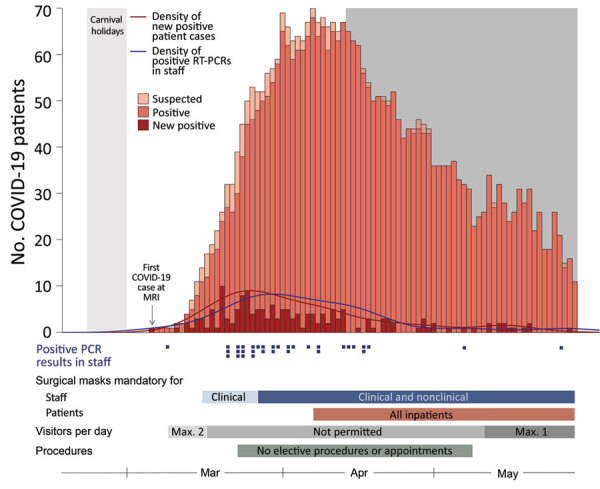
Prevalence and distribution of severe acute respiratory syndrome coronavirus 2 (SARS-CoV-2) infections in patients and staff at a university hospital in Munich, Germany. Shown is the number of all COVID-19 patients admitted to the hospital; the first COVID-19 patient was admitted on March 6, 2020. Light gray shading indicates dates of carnival holidays (February 22‒March 1, 2020); dark gray shading indicates dates of seroprevalence study (April 14‒May 29, 2020). Blue squares below graph indicate positive RT-PCR test results for SARS-CoV-2 RNA in university hospital staff. Bars below graph indicate densities of positive RT-PCR test results in staff (blue), new COVID-19 cases in patients (red), and limitations on number of visitors allowed and elective procedures and appointments (gray). COVID-19, coronavirus disease; Max., maximum; MRI, University Hospital Munich Rechts der Isar; RT-PCR, reverse transcription PCR.
